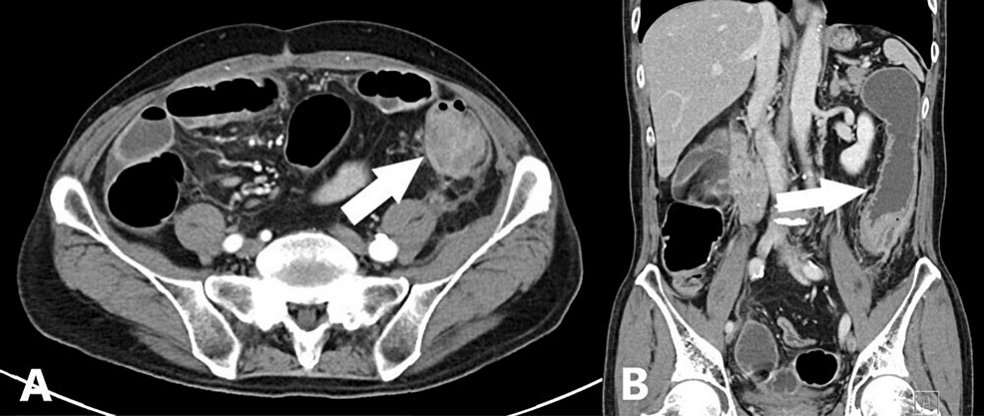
Abdominal contrast-enhanced CT scans, axial view (A) and coronal view (B) Wall edema (A, white arrow) and dilatation of the oral side (B, white arrow) were observed in the descending colon.
